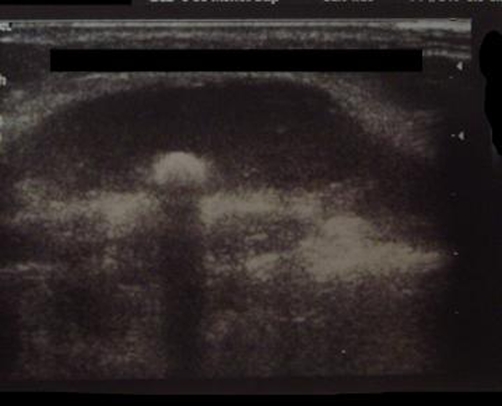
Ultrasound performed on day 1. Dislocation of the 3^rd^ sternal nuclei appear.
